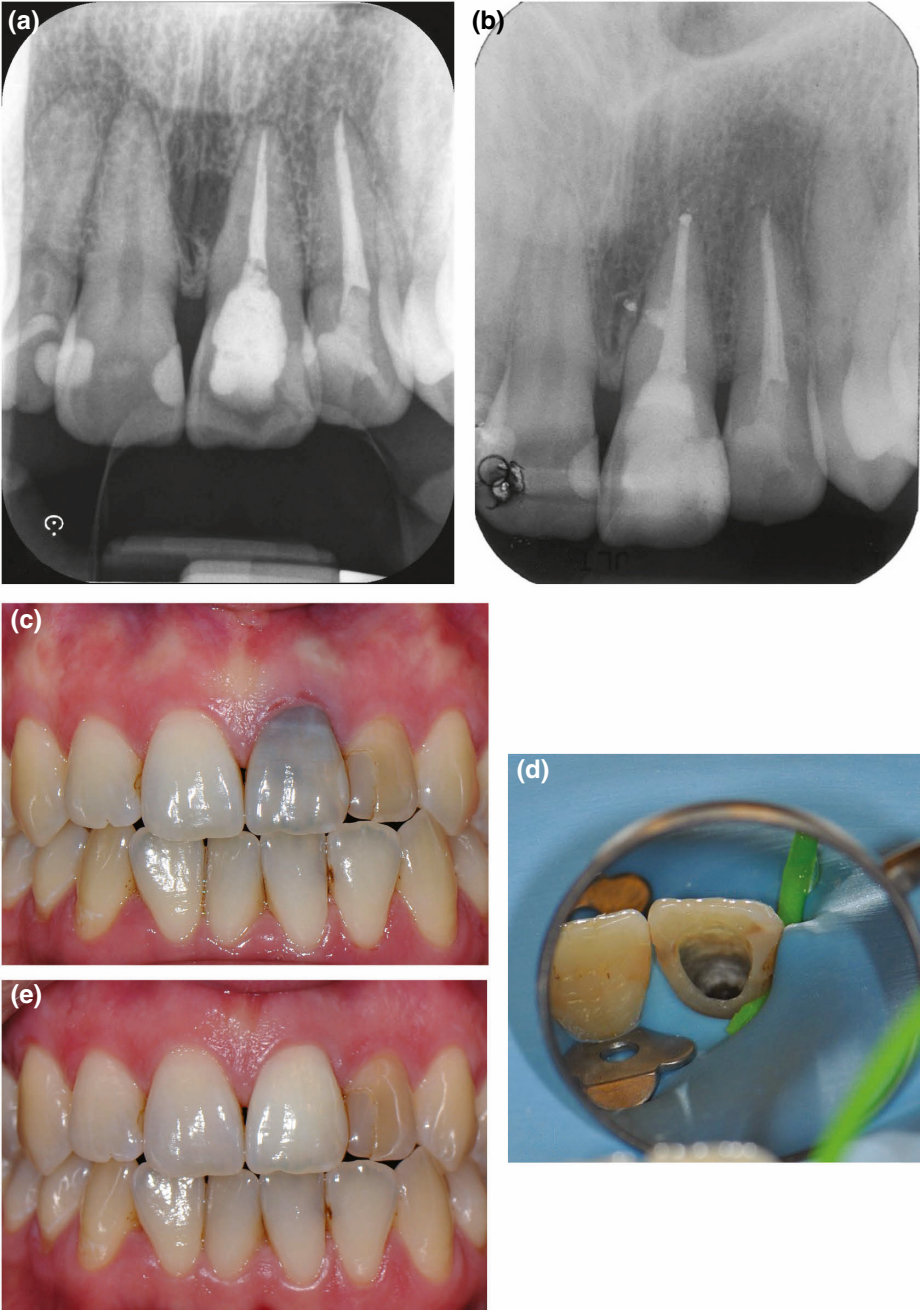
(a) A preoperative radiograph of a root filled left maxillary central incisor with pathos. (b) The tooth was successfully retreated. (c) A photograph showing the extent of discolouration. (d) A photograph of the open assess cavity. Bleaching utilized the walking bleach technique with sodium perborate (Endoprep bleach; PDS) mixed with water. (e) A post‐treatment photograph immediately post‐bleaching showing the bleached tooth having a lighter colour than the adjacent teeth. Courtesy of Dr Tom Fenelon
